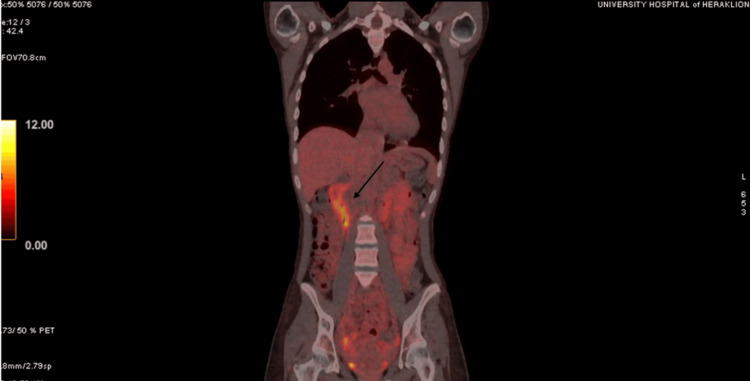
FDG-PET/CT scan FDG-PET: Fluorodeoxyglucose F 18 (18F-FDG) positron emission tomography; High metabolic activity observed in the duodenum and the small intestine (maximum standardized uptake value or SUVmax 7.26).
